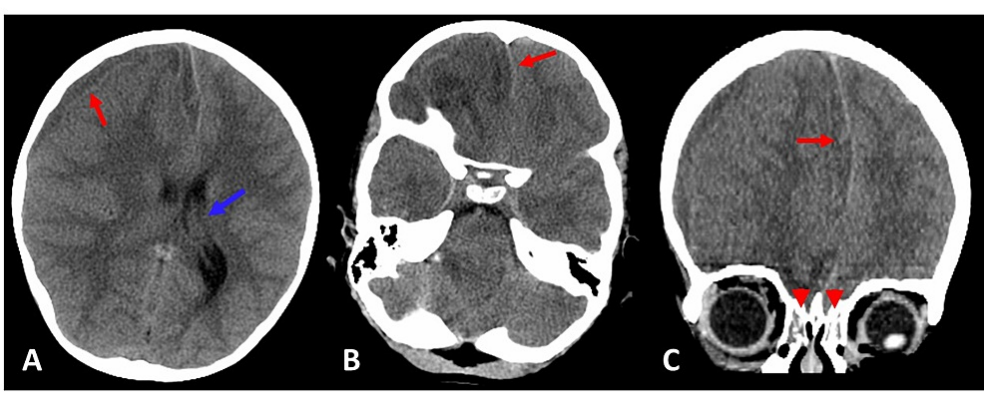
Computed tomography brain without contrast: hypodense right frontal temporal subdural hematoma extending along the anterior falx measuring 8 mm in greatest dimensions and causing right to left midline shift measuring 7 mm (red arrows). (A) Transverse view showing dilatation of the left lateral ventricle including the temporal horn concerning for mild entrapment (blue arrow). (B) More caudal transverse view showing midline shift (red arrow). (C) Coronal view showing midline shift (red arrow); there was also bilateral ethmoid sinusitis (red arrowheads).
